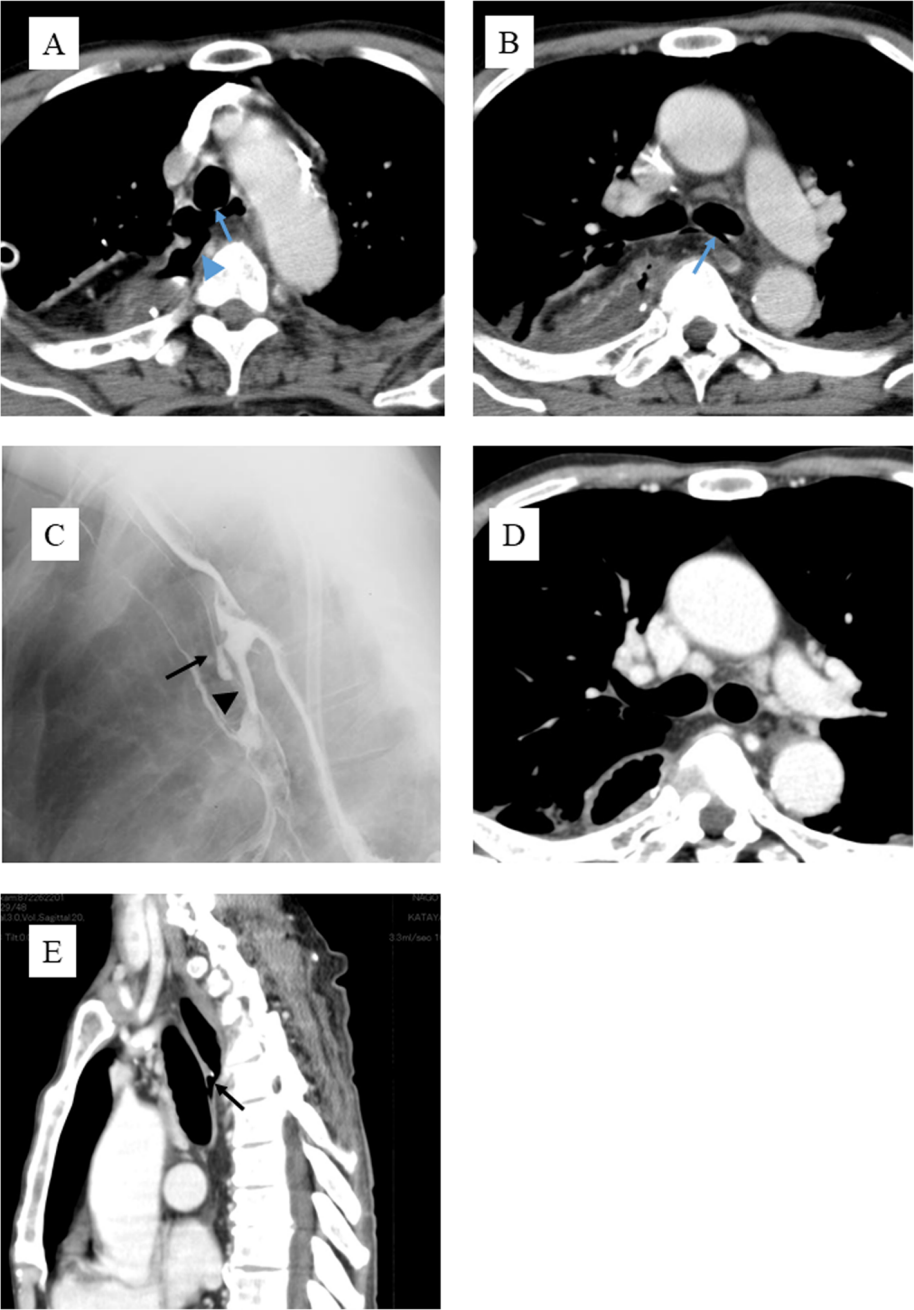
On postoperative day 7, computed tomography (CT) revealed anastomotic leakage (blue arrowhead) and a tracheal fistula (blue arrow) (a). On postoperative day 7, a left main bronchial fistula was observed (blue arrow) (b). On postoperative day 49, an upper gastrointestinal series showed a fistula within the trachea (black arrow) and a fistula within the left main bronchus (black arrowhead) (c). CT (postoperative day 117) revealed that the left main bronchial fistula was closed by covering it with omentum (d), and the GTF was open (black arrow) (e)
